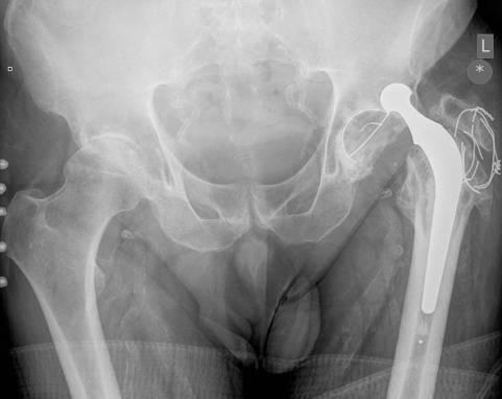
Plain X-ray of the pelvis showing a dislocated left hip with a broken wire in the left hip.
